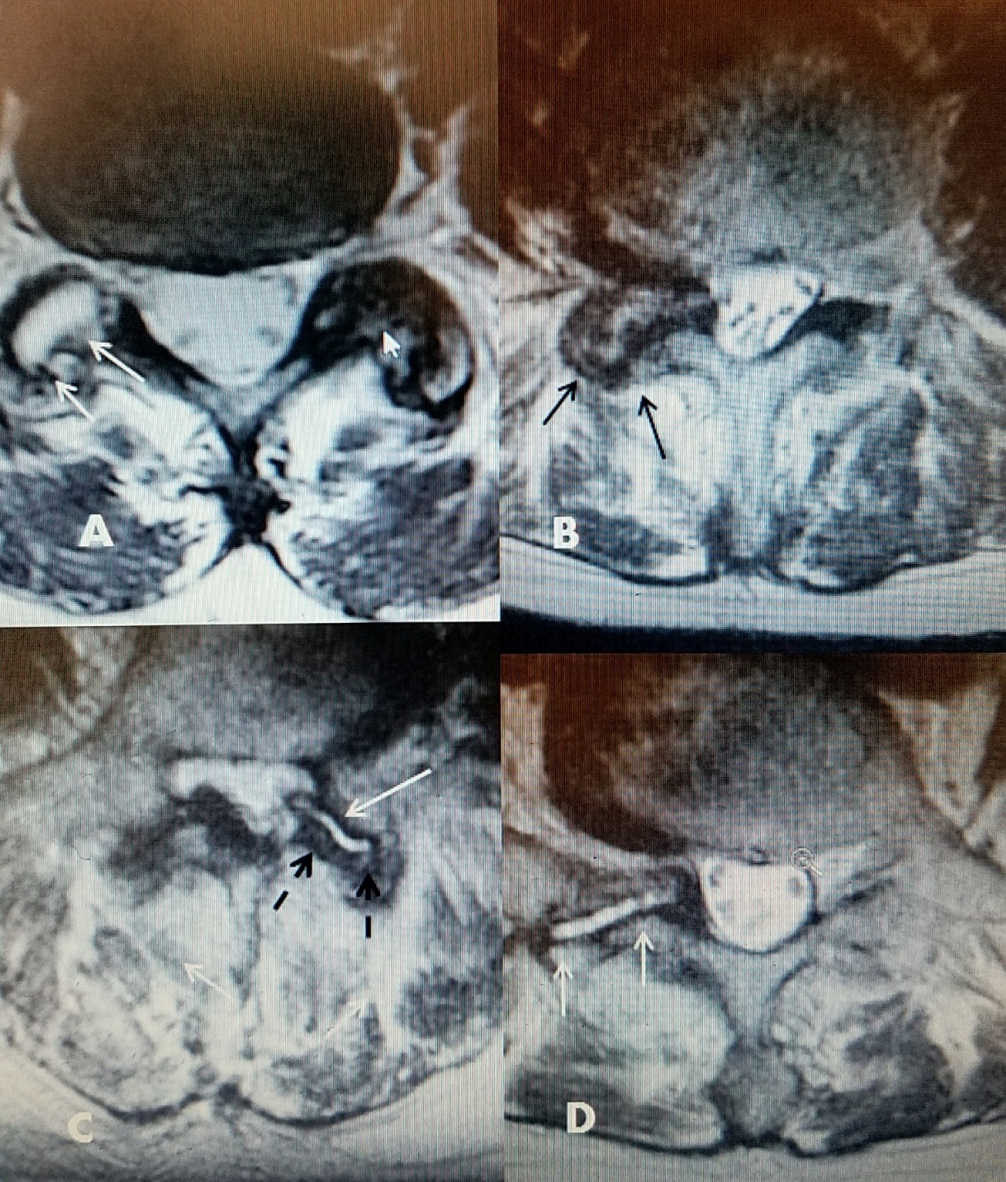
Axial MRI images of the lumbar facet joints showing fluid, osteoarthritis, and facet capsule enlargement A: L5-S1 bilateral abnormal facets. There is hypodense fluid signal in one facet joint (two white arrows) with an enlarged joint space. The hypertrophied facet is on the opposite side with reactive bone overgrowth (small solid white arrow). B: L4-5 marked degenerative facet hypertrophy (solid black arrows), causing severe foramena and lateral recess stenosis. C: L4-5 unilateral facet fluid and enlargement of capsule into the ventral spinal canal, causing lateral recess stenosis (white arrow). Hypertrophied posterior capsule and bone (dashed black arrows). There is thickening of the ligamentum flavum posteriorly on the opposite side. D: Unilateral enlarged L5-S1 facet with fluid (white hypodense signal on T2 magnetic resonance imaging (MRI)), causing foramena and lateral recess stenosis (solid white arrows).
